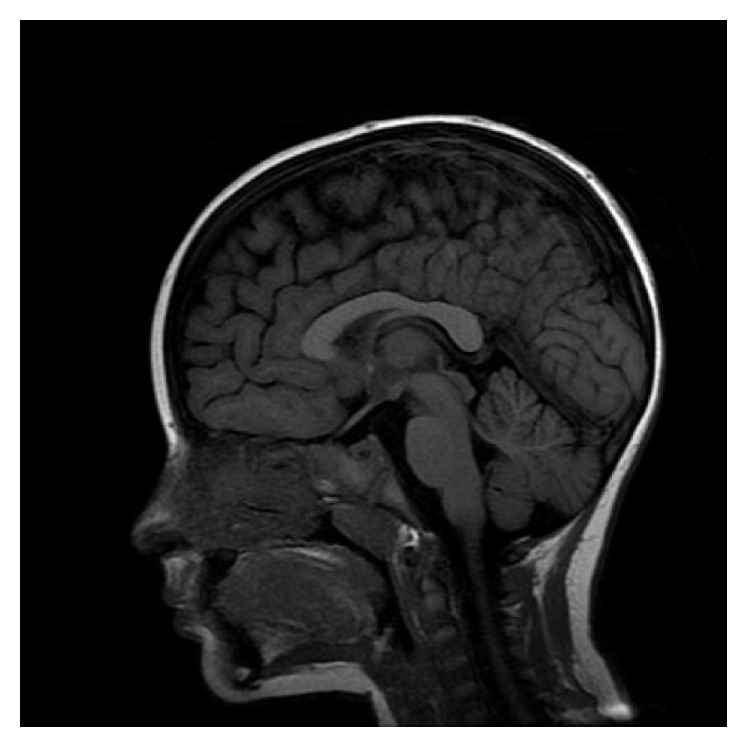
Brain MRI. Sagittal T1-weighted image demonstrating intact corpus callosum.
